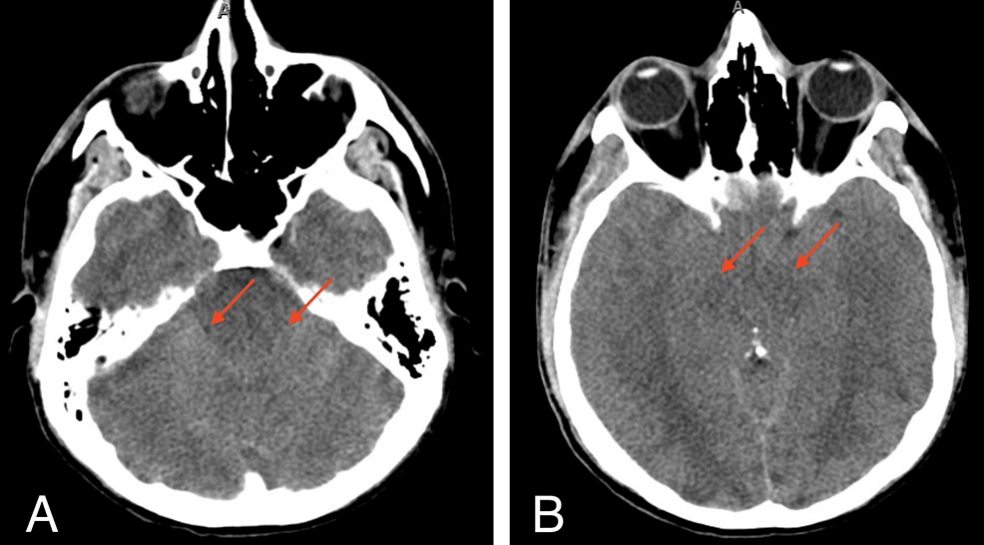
Axial head CT demonstrating diffuse cerebral edema and effacement of basal cisterns (red arrows), most consistent with severe edema and herniation
